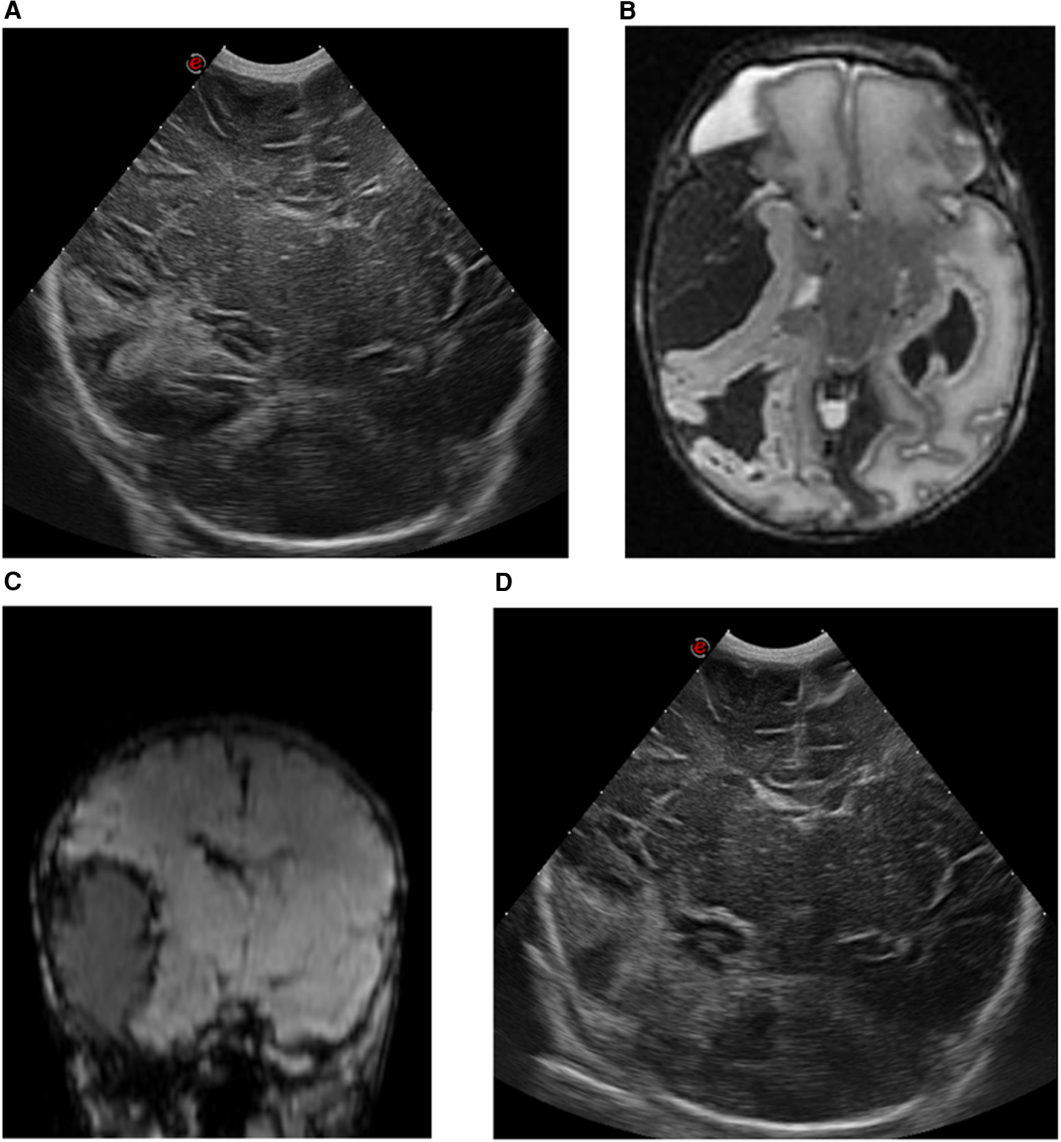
cUS and MRI findings of case 1. (A) cUS coronal view, showing a right-sided echogenic lesion temporo-occipital with midline shift to the left. (B) MRI T2-weighted sequence, axial view, and (C) MRI susceptibility-weighted imaging sequence, coronal view, showing an intra-axial hemorrhage in the right temporo-occipital region with an extra-axial component in the frontotemporal region, with a maximal thickness of 32 mm. This causes a mass effect on the right temporal lobe with midline shift of 5 mm to the left. (D) cUS coronal view, after surgical intervention.
